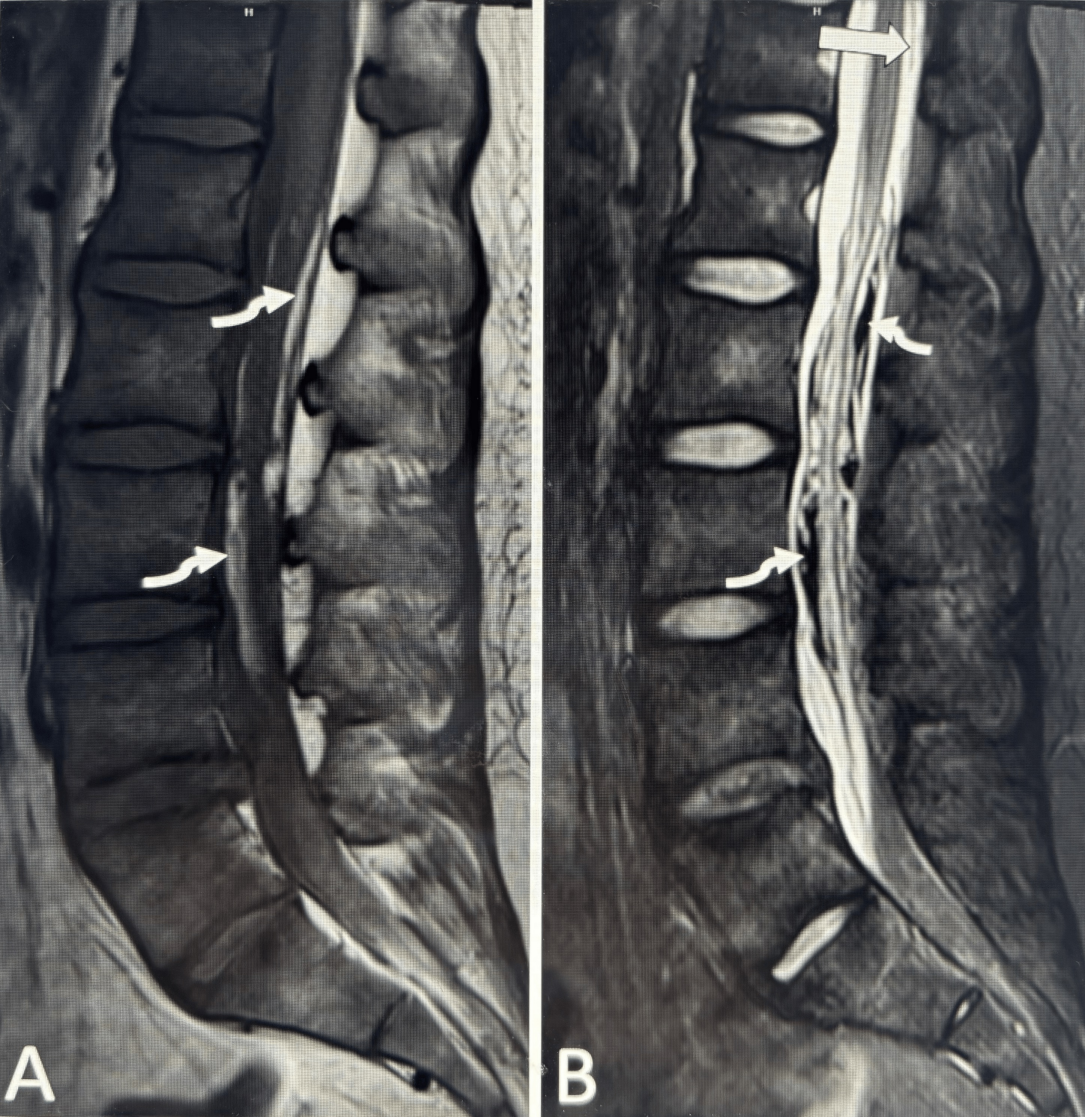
Lumbar spine MRI (Patient 2) Lumbar spine MRI of Patient 2, performed before EBP. Sagittal T1-weighted (A) and sagittal T2-weighted (B) images of the lumbar spine reveal T1 hyperintense and T2 hypointense signals within the thecal sac related to blood products from a recent traumatic LP (curved arrows). There is a thin layer of fluid dorsal to the dura at the T12 level (solid arrow), likely representing epidural fluid from a CSF leak. LP: lumbar puncture; CSF: cerebrospinal fluid
